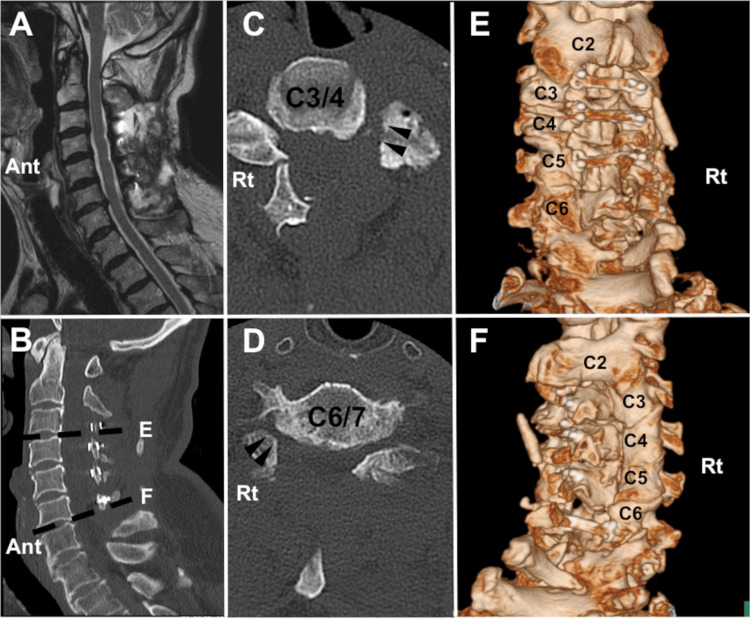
Radiographic images after the operation (case 1). Cervical canal stenosis was resolved after the operation (A: sagittal magnetic resonance image, B: sagittal computed tomography image). Posterior decompression is achieved after foraminotomy in the left C3/4 (C) and right C6/7 (D) levels. Left laminoplasty is performed from C3 to C5 (E), while right laminoplasty is performed at the level of C6 (F) (Ant: anterior, Rt: right)
